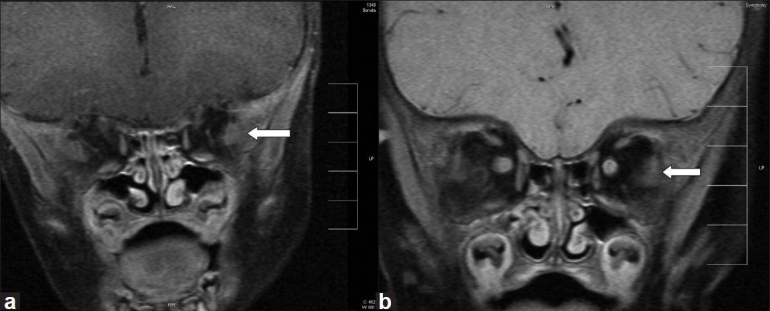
MRI orbits of patients 2 (a) and 3 (b). T1-weighted images showing remarkable atrophy of all extraocular muscles except lateral rectus (white arrow)
